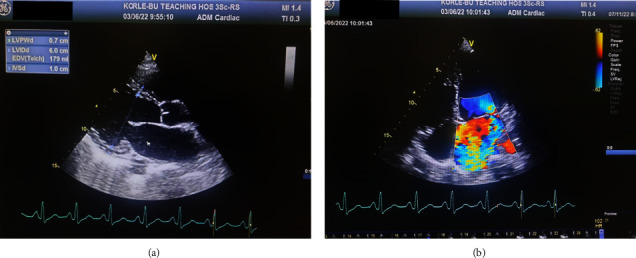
Echocardiogram 5 weeks post imatinib: (a) 2D parasternal long axis view showing dilated left ventricle, left atrium and thickened mitral valve leaflets. (b) 2D apical 4-chamber view showing severe mitral regurgitation on colour doppler.
